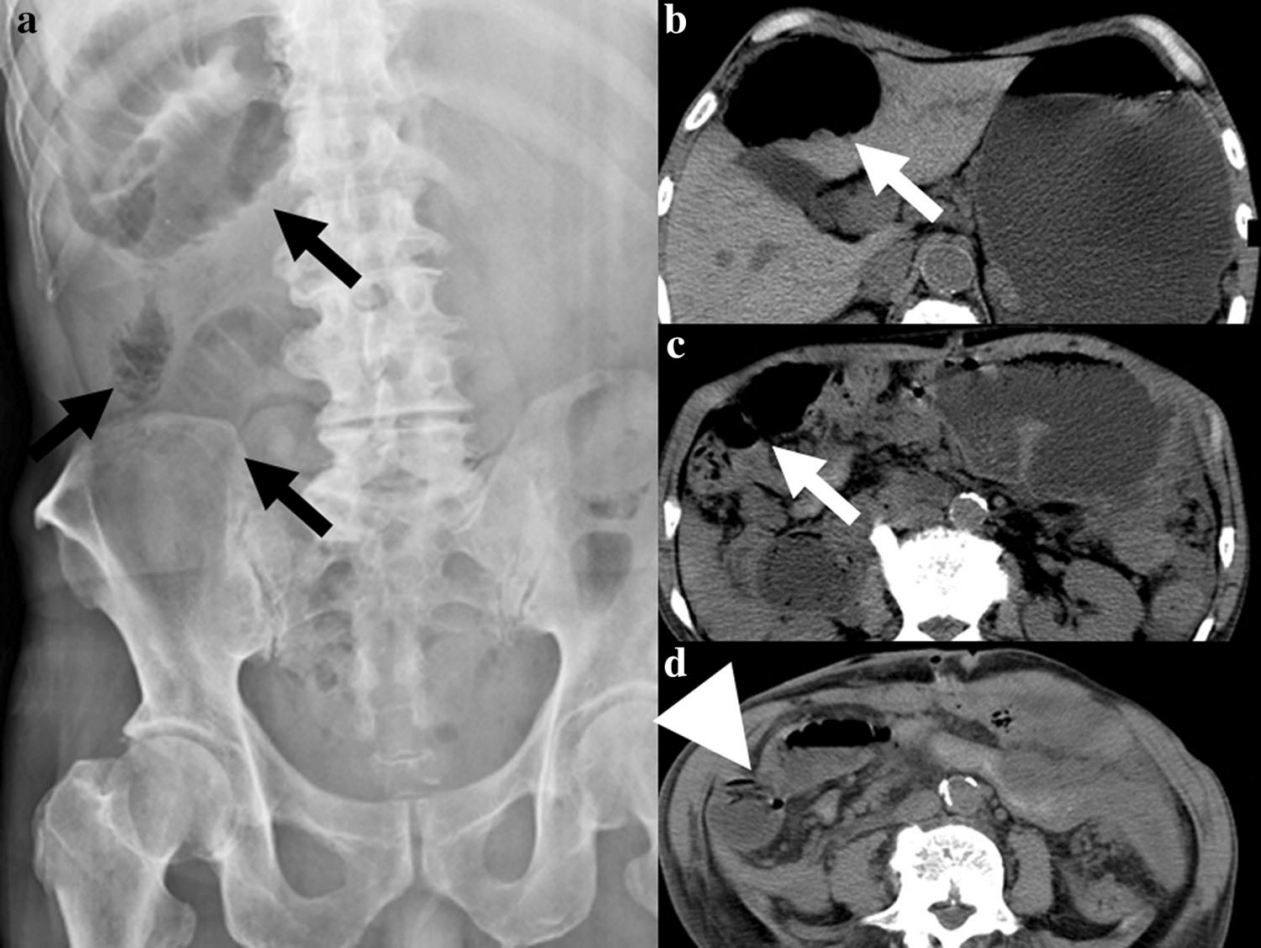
Findings of abdominal X-ray and computed tomography taken at the onset of bowel strangulation. a Abnormal intestinal gases (*black arrows*) observed by abdominal X-ray suggested formation of several intestinal loops. b Dilated intestine was observed in the right upper abdomen. c, d Dilatation of the intestine was tapered (*white arrow*) and disrupted by the severe caliber change (*white arrowhead*). These findings suggested that bowel strangulation was caused by the torsion of the intestinal loop
